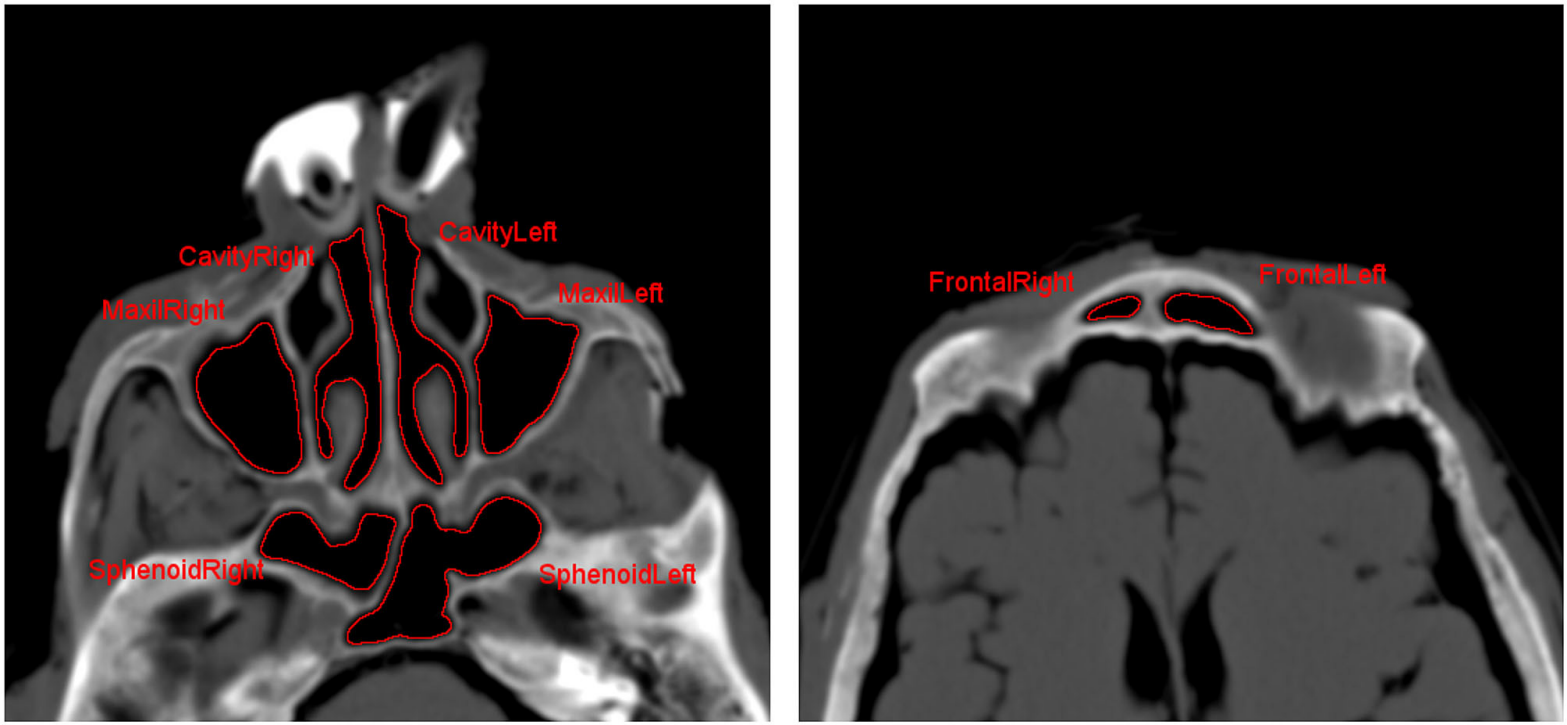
Axial CT scan for placement of regions of interest (ROIs) in nasal cavity as well as in the different paranasal sinuses.
